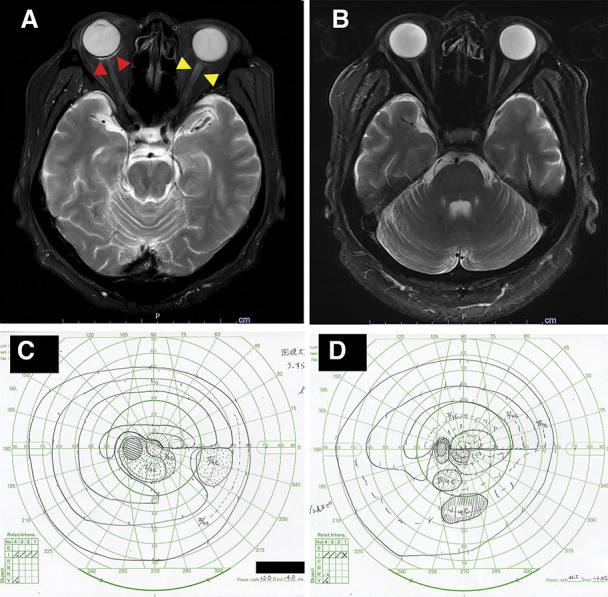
Magnetic resonance imaging and kinetic perimetry of optic neuritis at onset and after treatment. A. MRI at onset. Fat-saturated T2-weighted MRI shows hyperintensity along the sclera in the right retrobulbar (*red arrowheads*), suggesting inflammation, and a longitudinal enhancing lesion of the left orbital nerve (*yellow arrowheads*), suggesting optic neuritis. No findings are suggestive of meningitis. B. MRI after treatment. The T2-weighted fat-saturated image shows the subtle residual high signal along the sclera in the right retrobulbar and the normal signal near the papilla of the left optic nerve. C. GP at onset. Kinetic perimetry shows the lower horizontal hemianopia. D. GP after treatment. The lower horizontal hemianopia has narrowed.
